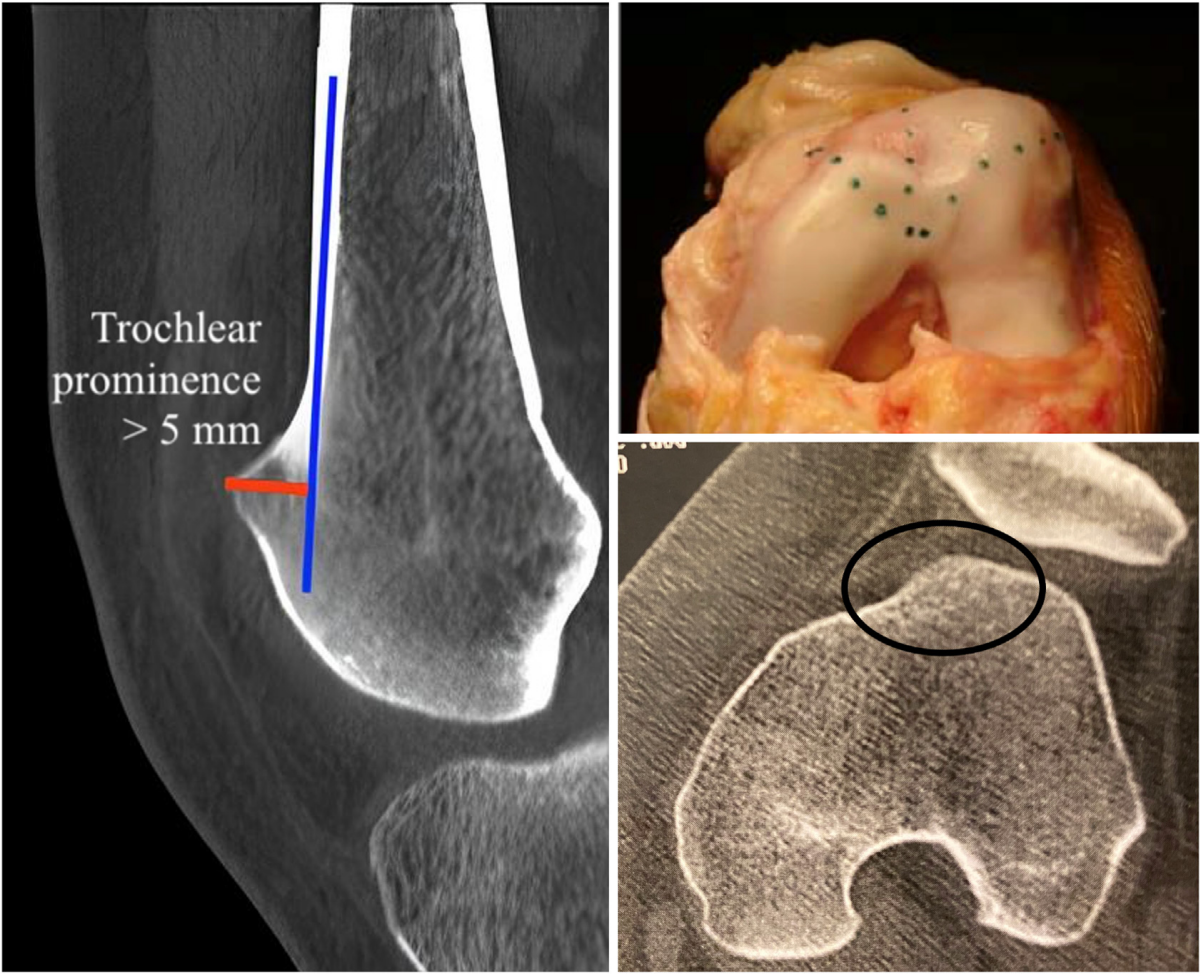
Trochlear prominence greater than 5 mm, as seen on computed tomography in sagittal and axial views, and during surgery (left knee). Blue line indicates anterior cortical and orange oval lines indicate troclear proeminence.
